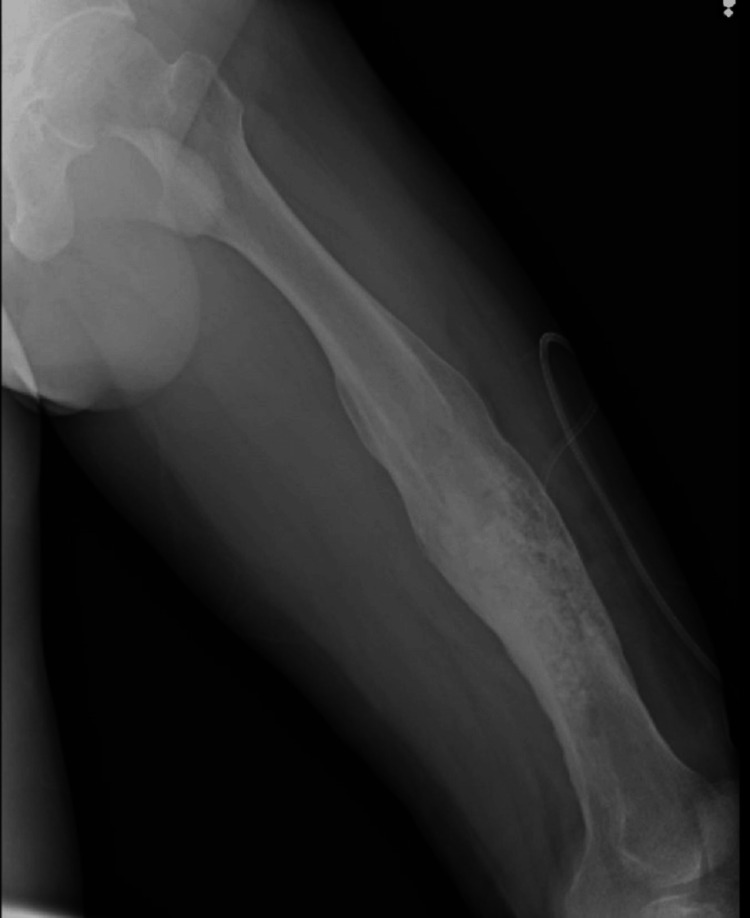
Postoperative radiograph showing the clearance of sequestrum
